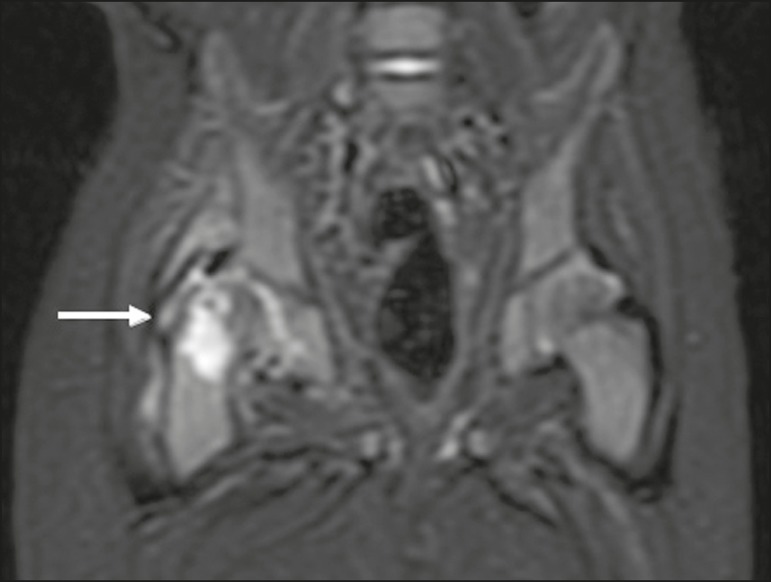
MRI of the hip. STIR coronal sequence showing mild effusion in the right hip joint, with synovial thickening and hyperintense signal/edema in the adjacent planes of the capsule of the muscle. Note the irregular lesion, with a hyperintense signal in a T2-weighted sequence, in the right femoral head and neck, which is suggestive of a focus of osteomyelitis/intraosseous abscess.
